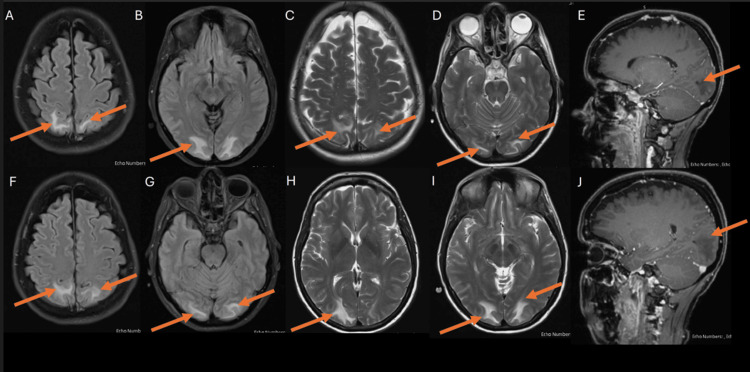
MRI of the head and spine with IV gadolinium showing bilateral cortical and subcortical areas with white matter extension, appearing relatively symmetrical, with high signal intensity (arrows) on T2-weighted imaging (C, D, H, I) and FLAIR (A, B, F, G), corresponding to low signal intensity on T1-weighted imaging, predominantly in the parieto-occipital lobes. Susceptibility-weighted imaging showed no blooming. No definite post-contrast enhancement was observed (E, J). Mild mass effect was noted on the adjacent sulci and occipital horns. Findings are consistent with PRES. PRES, posterior reversible encephalopathy syndrome
